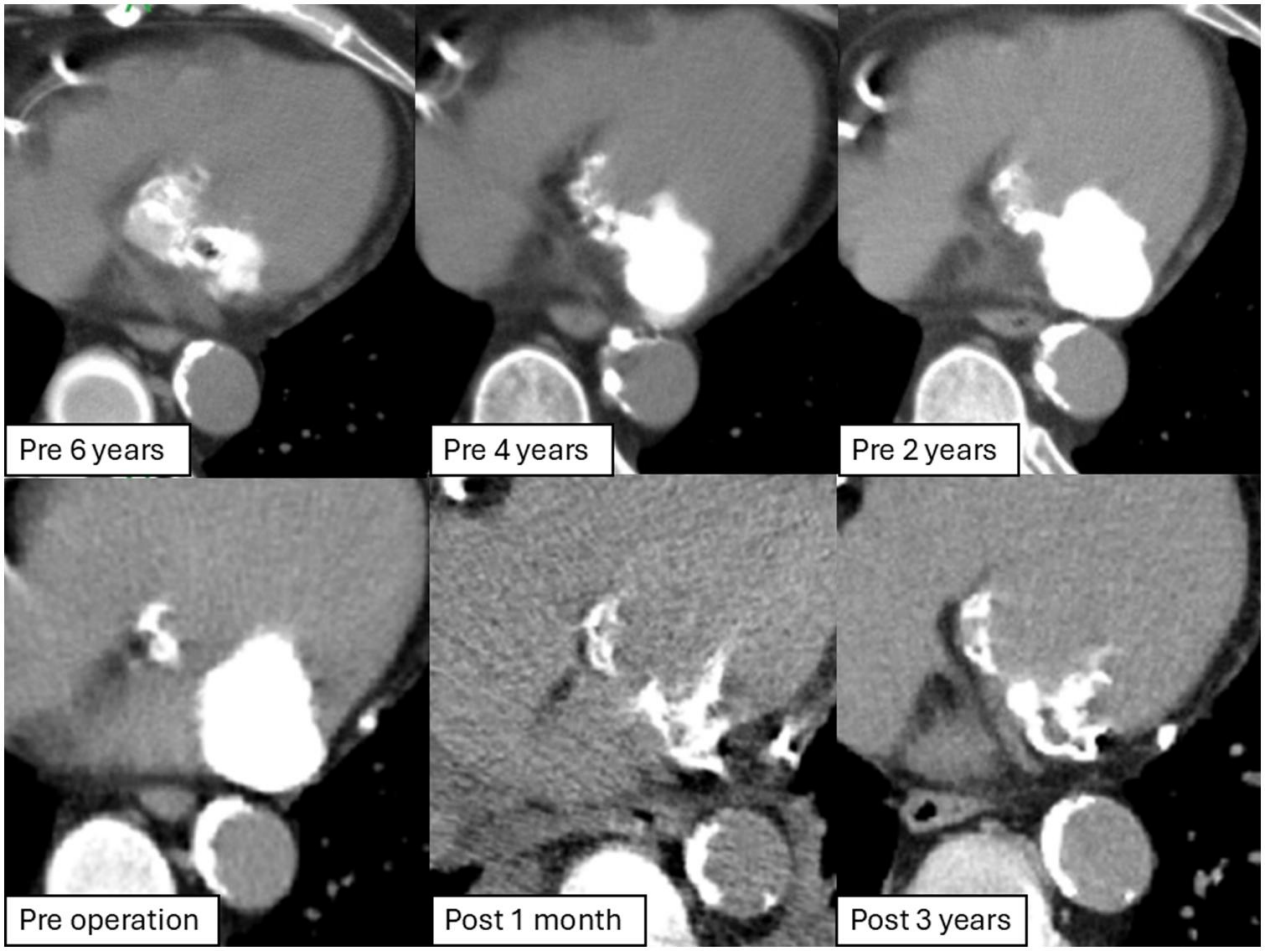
Computed tomography images. CT findings from 6 years preoperatively to 3 years postoperatively. They showed an increase in size of the mass over time, 38×21 mm preoperatively. Postoperatively, there was no evidence of recurrence
